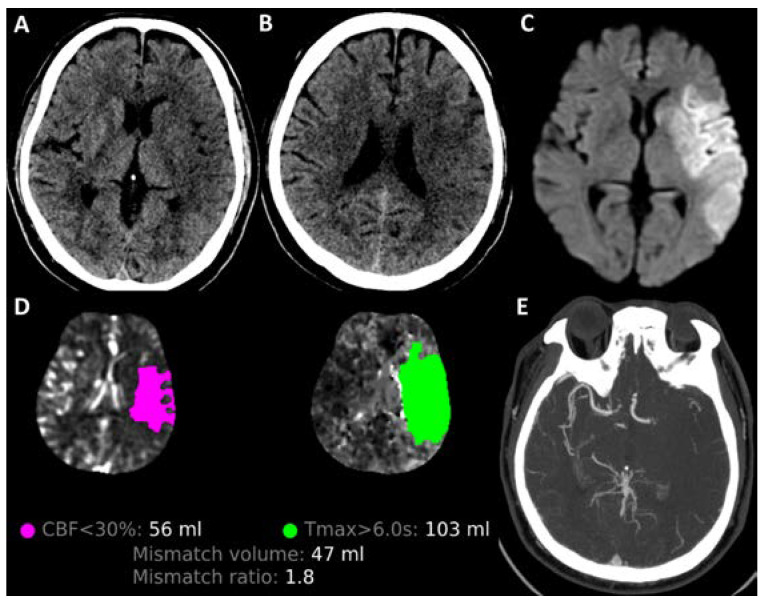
A large infarct core volume (LICV) stroke is demonstrated in this figure. There is a left middle cerebral artery territory (MCA) infarct with loss of grey–white differentiation in the left caudate, internal capsule, insula, M2 (A), as well as left M5 and M6 regions (B), giving an ASPECTS of 4. MRI brain shows corresponding DWI hyperintensities in the left MCA territory (C). CT perfusion imaging processed by RAPID software (v 1.3.3) shows the ischaemic core and perfusion deficit. The ischaemic core (pink) is 56 mL while the tissue at risk of infarction (green) is 103 mL, giving a large mismatch ratio of 1.8 (D). CT vessel angiogram shows a left M1 large vessel occlusion (E).
